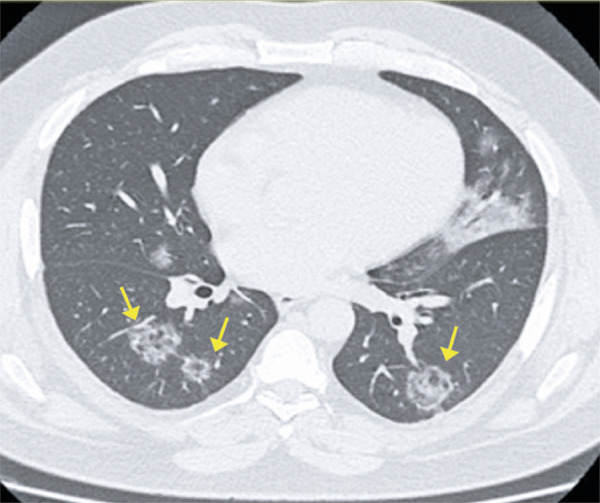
A 43-year-old patient with COVID-19, presenting shortness of breath, fever and cough for eight days. Computed tomography showing reversed halo sign in the lower lobes
